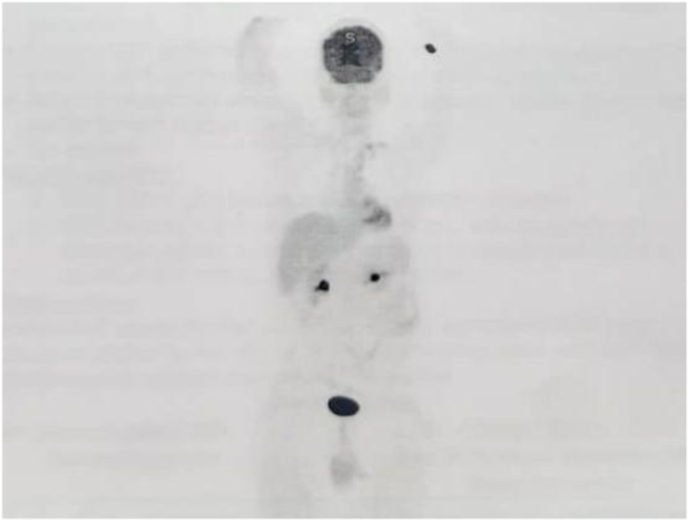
18F-fluorodeoxyglucose positron emission tomography (FDG–PET). Done on the latest follow-up appointment denoting a clear study, with the absence of any local recurrence or distant metastases.
